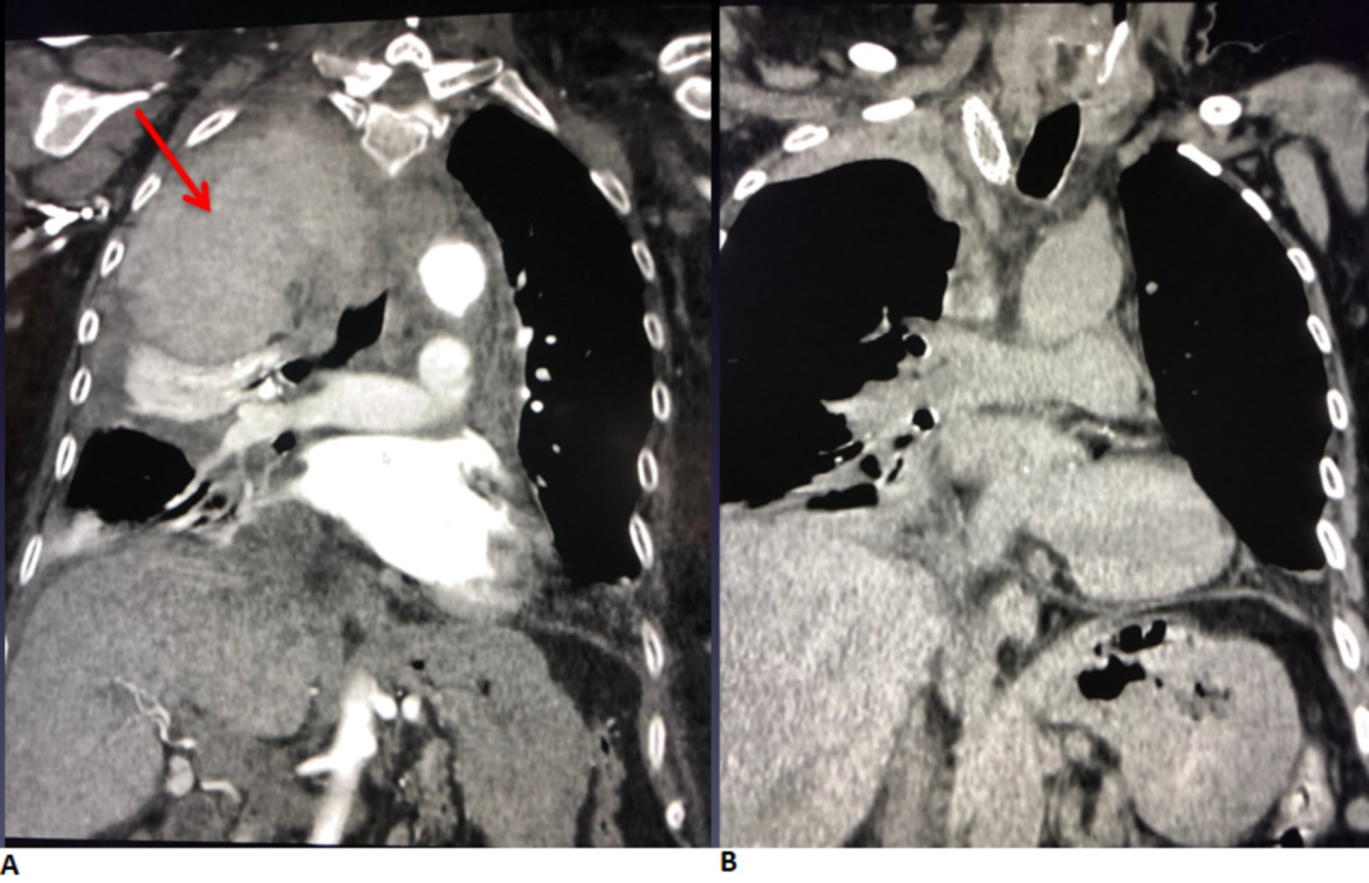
(A) Coronal CT angiogram demonstrating extrapleural hematoma compressing the right lung apex. (B) Coronal CT post CT-guided drainage of extrapleural hematoma.
